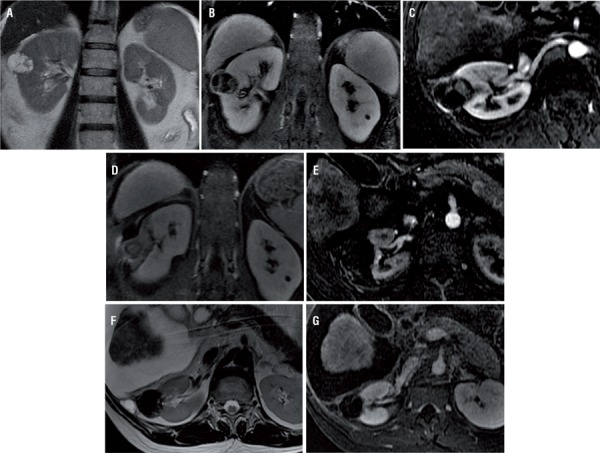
(a-g) 48-years old man with a Bosniak IV lesion. Coronal T2-weighted (a), Coronal T1-weighted gradient echo axial MRI sequences with fat suppression (b) and coronal subtraction image (postcontrast arterial phase data - precontrast data) (c) show a cystic renal mass with thickened enhancing septa and a small solid component. Coronal T1-weighted gradient echo axial MRI sequences with fat suppression (d) performed immediately after the procedure show complete ablation of cystic lesion and no measurable enhancement within ablation zone. Axial subtraction image (postcontrast arterial phase data - precontrast data) (e) three months after the procedure show no enhancement and no recurrent of the neoplasm. Axial T2-weighted (f) and axial subtraction image (postcontrast arterial phase data - precontrast data) (g) 3 years after the procedure show ablation changes in the right kidney, without residual enhancement to suggest recurrent neoplasm.
